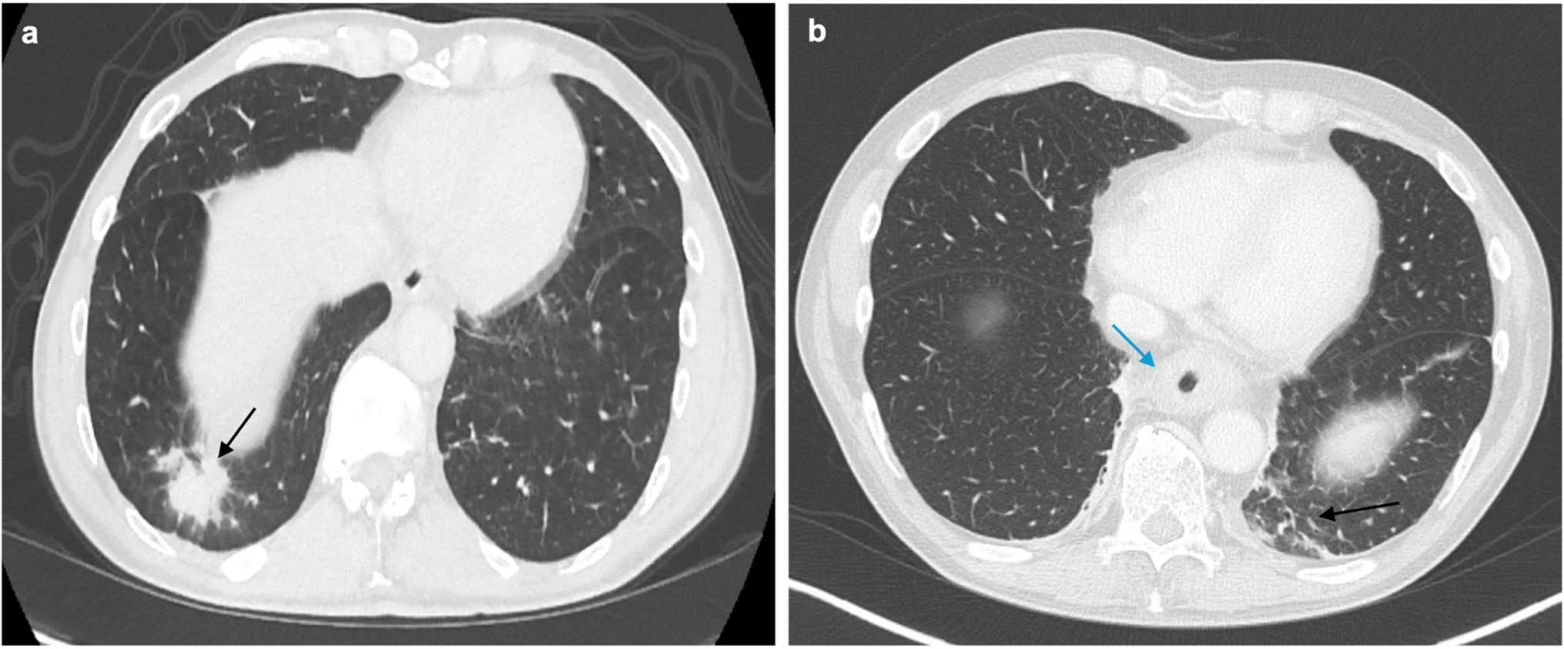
CT scan of the chest (a, b) demonstrates patchy consolidative opacities with ground-glass attenuation and fibrosis (black arrows) which are characteristic findings of radiation-related organizing pneumonia. There is also radiation-induced wall thickening of the esophagus (blue arrow)
